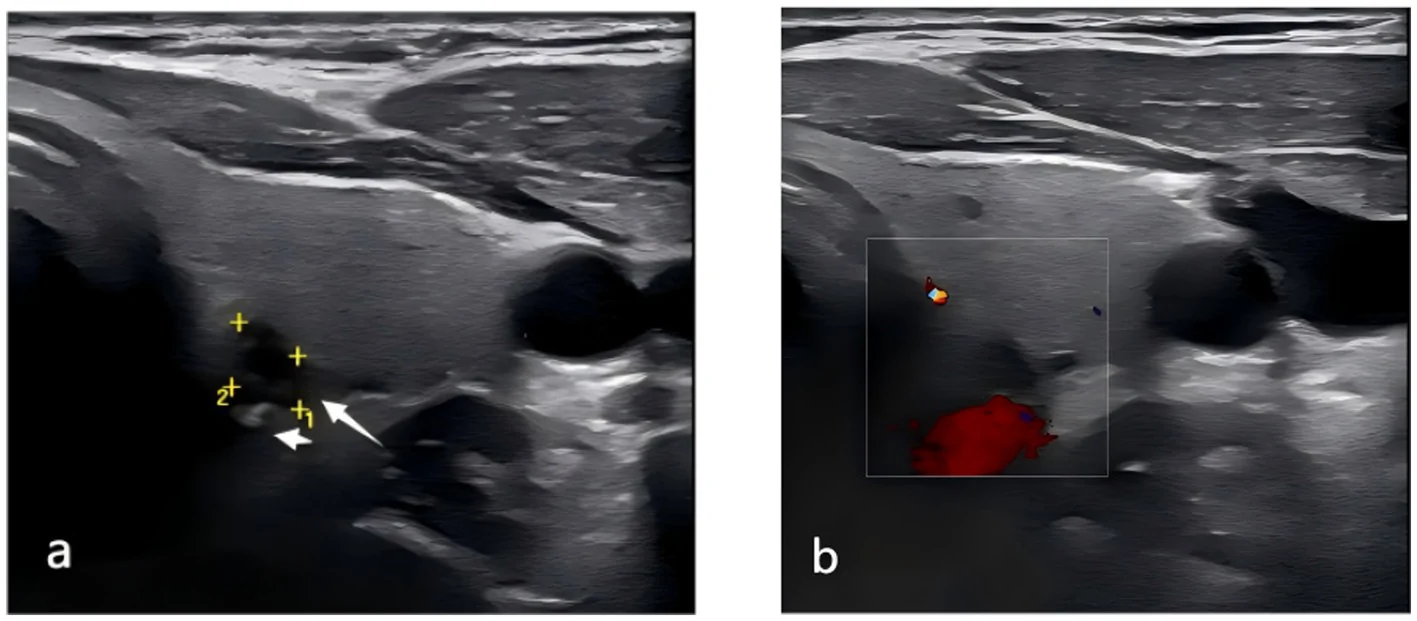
Thyroid gray-scale ultrasound examination and color Doppler flow imaging of a 38-year-old male patient. (a) The gray-scale ultrasound examination revealed a hypoechoic nodule located in the posterior-medial part of the left lobe of the thyroid gland (indicated by the arrow), with a size of 5.6 × 3.8 mm. The edge of the nodule was clear, and there were punctate hyperechoic areas (indicated by the short arrow) in the posterior-medial part. (b) The color Doppler imaging showed no vascular distribution within the nodule.
